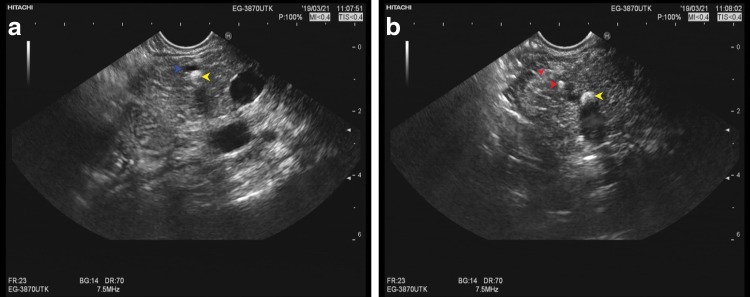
(a, b) Endoscopic ultrasound showing low pancreatic echoes, the internal echoes were uniform. Pancreatic duct in the head and neck of the pancreas showed multiple stone shadows (yellow arrow: pancreatic stones; red arrow: parenchyma calcification; and blue arrow: pancreatic duct).
